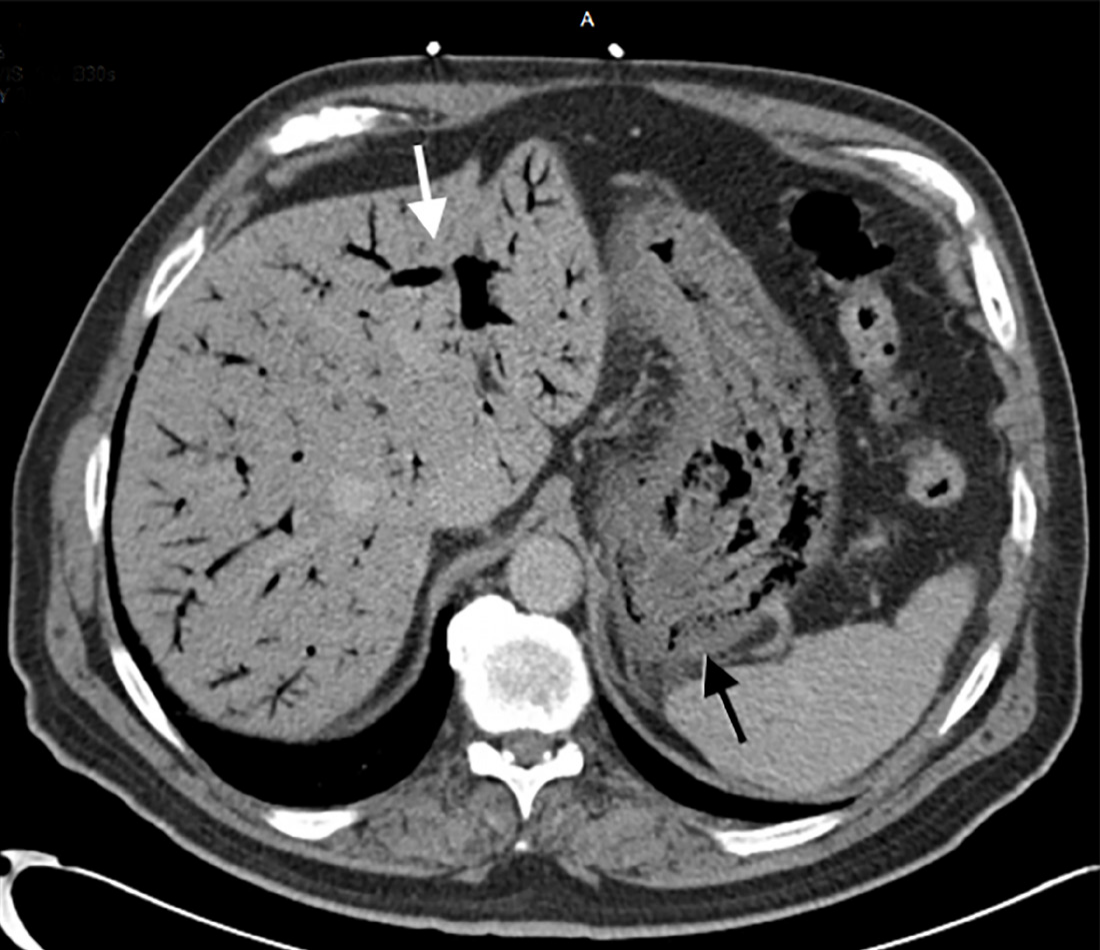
Computed tomography of the abdomen and pelvis without contrast reveals pneumatosis in the walls of the stomach (black arrow) and extensive portal venous gas (white arrow).
